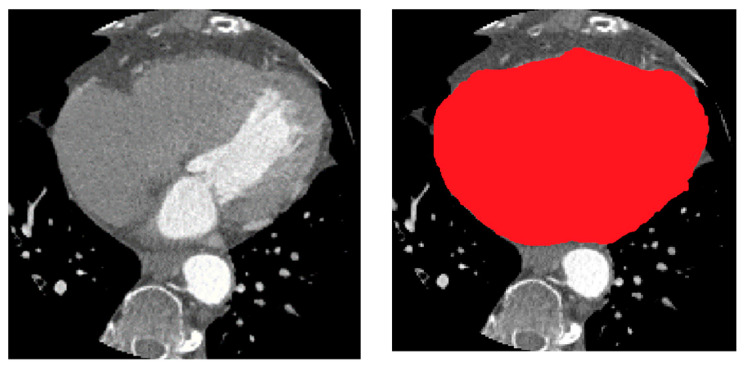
Example of heart segmentation from target CTA.
